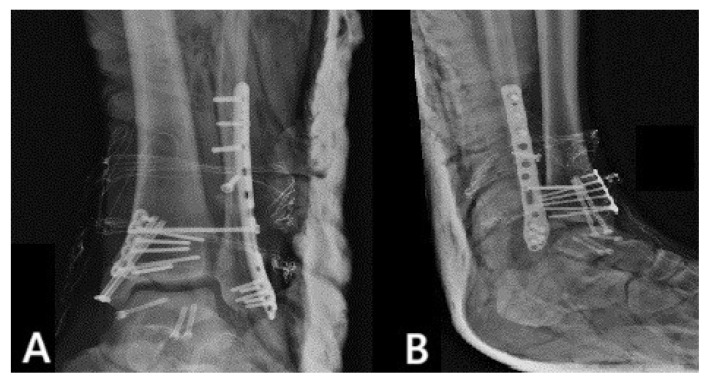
(A) Anteroposterior and (B) lateral immediate postoperative X-ray views of the ankle showing ORIF of the bimalleolar fracture and ORIF of the talar body fracture.
